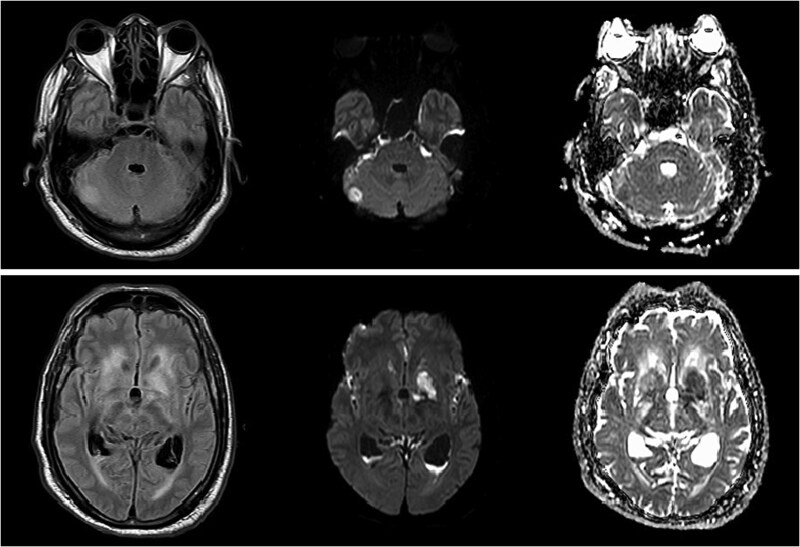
Follow-up brain MRI revealing acute infarcts in the right cerebellum and left basal ganglia.
